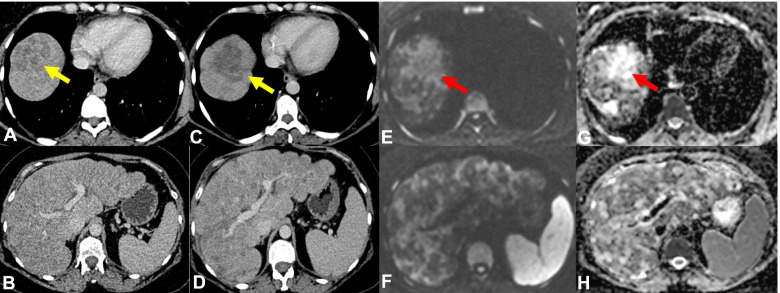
WBMRI better characterising diffuse liver disease. 43-year-old female with patient with ER- PR- positive HER-2 negative metastatic breast cancer to the nodes, lung and liver was being monitored with CT during chemotherapy treatment. Follow up CT following 3 cycles demonstrates enlarging and more confluent liver lesion at the liver dome (C – yellow arrow) compared to prior CT (A—yellow arrow) and a greater number of lesions elsewhere within the liver suggesting worsening disease (D) when compared to prior CT (B) however tumour markers were improving on treatment. The increasingly irregular liver contour suggests pseudocirrhosis (D). WBMRI was performed to further evaluate the CT findings. The high b value (b900) DWI images (E) and corresponding ADC map (F) show diffuse disease with some lesions demonstrating high ADC values representing low cellularity/treated disease and others demonstrating low ADC values representing high cellularity/active disease in keeping with a mixture of treated and active disease, indicating a favourable response to treatment in some areas
